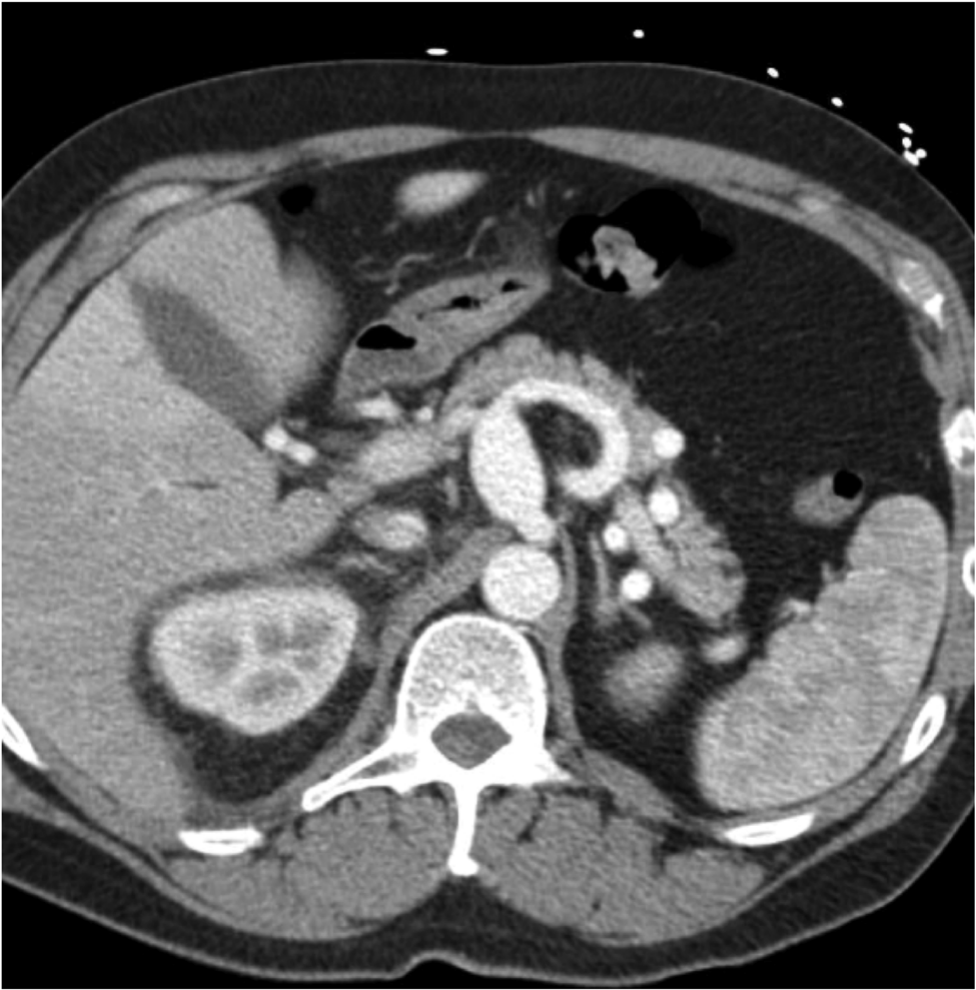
Preoperative CT scan of hepatic artery aneurysm (CHA).
